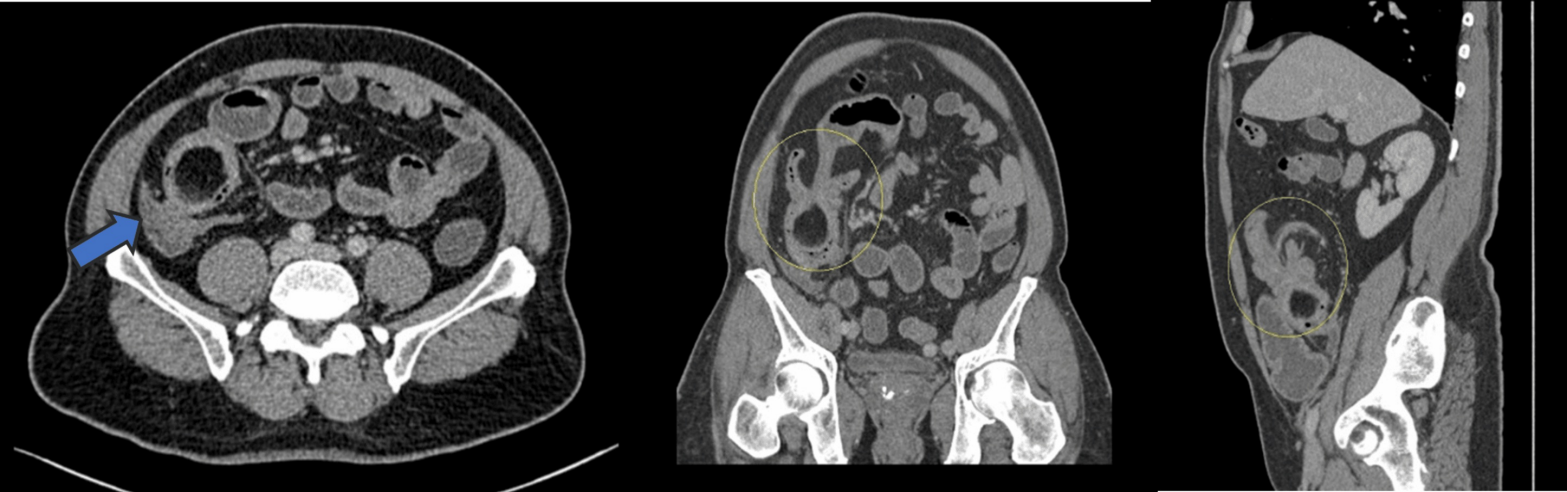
Axial (left), coronal oblique (middle), and sagittal (right) contrast-enhanced CT images showing a round fat-density mass in the distal ileum, with invagination of the ileum and resultant proximal small bowel distension, due to intussusception of ileal lipoma.
